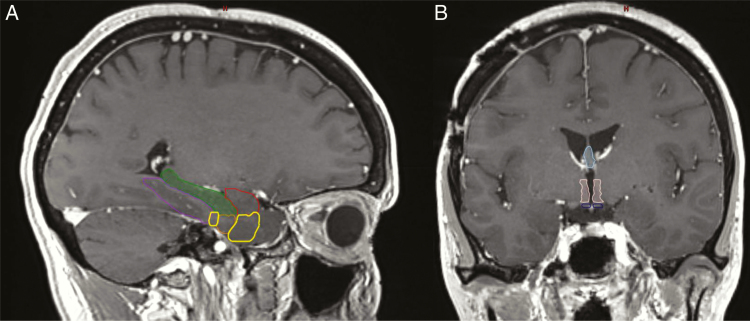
(A) Sagittal MRI demonstrating the anatomy of the medial temporal lobe structures: the amygdala (red), hippocampus (green), parahippocampal cortex (purple), perirhinal cortex (yellow), and the entorhinal cortex (orange). (B) Coronal MRI demonstrating the fornix (light blue), hypothalamus (light pink), and mammary bodies (dark blue).
